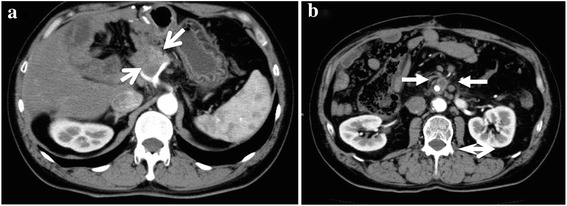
About 1 year after the hepatectomy, the abdominal lymph node metastases around the celiac artery (a; arrow) and along the superior mesenteric artery (b; arrow) are detected by the abdominal computed tomography
